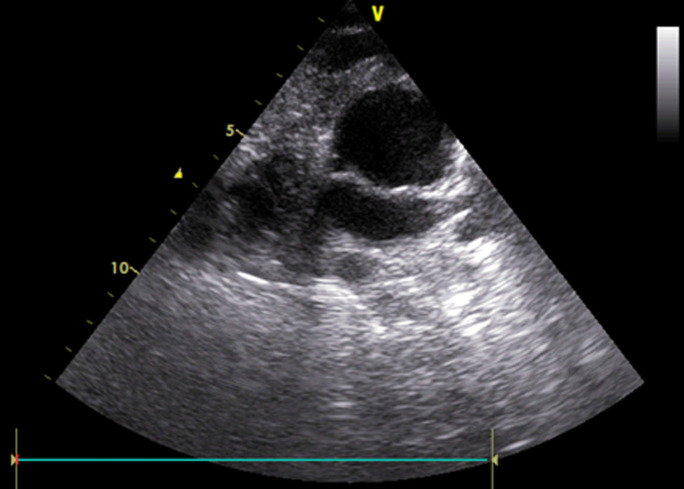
trans-thoracic echocardiogram revealed at admission an important distention of the right ventricle
